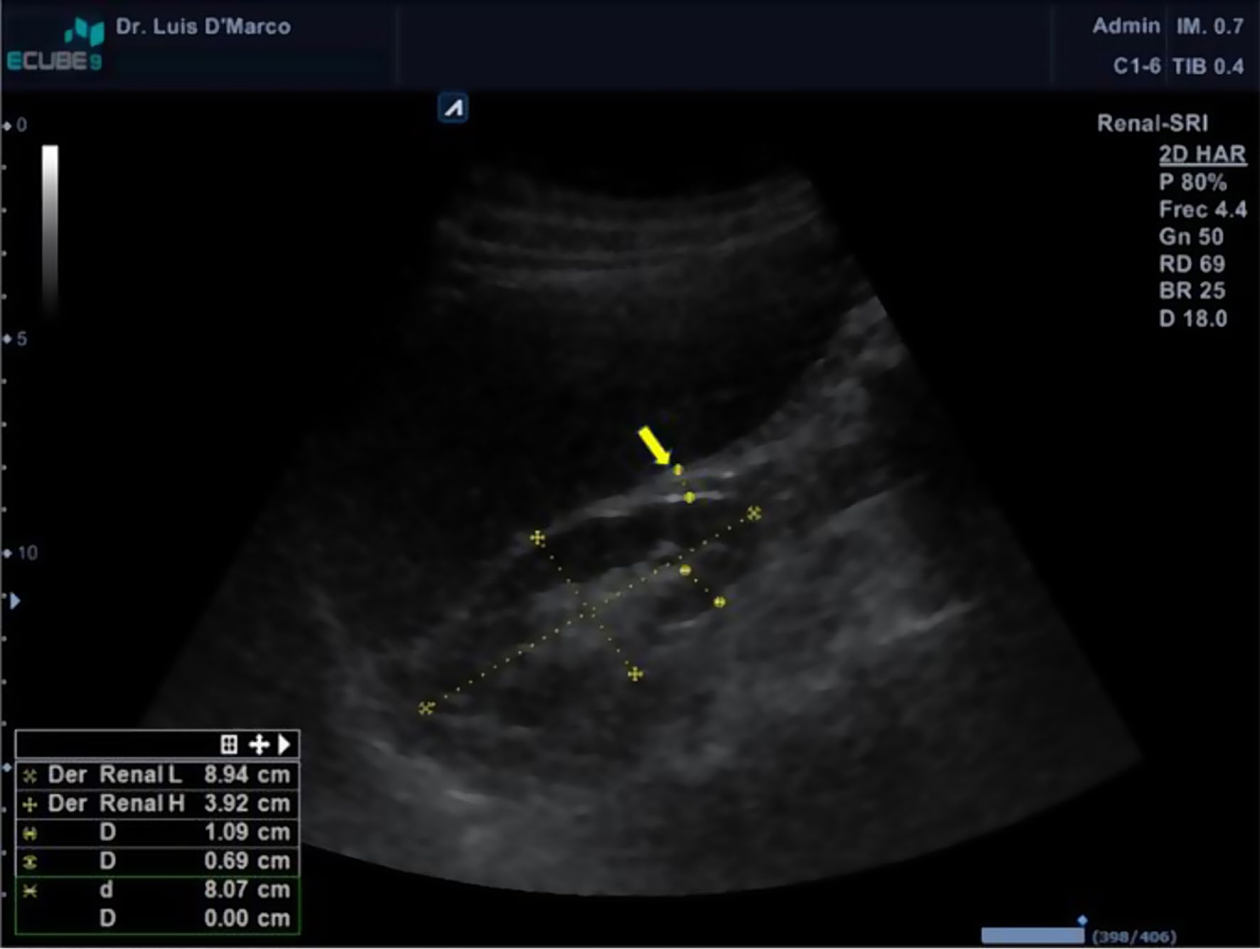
Imaging of the perirenal adipose tissue measurement by ultrasound. The arrow shows the adipose tissue thickness in the right kidney [21]
